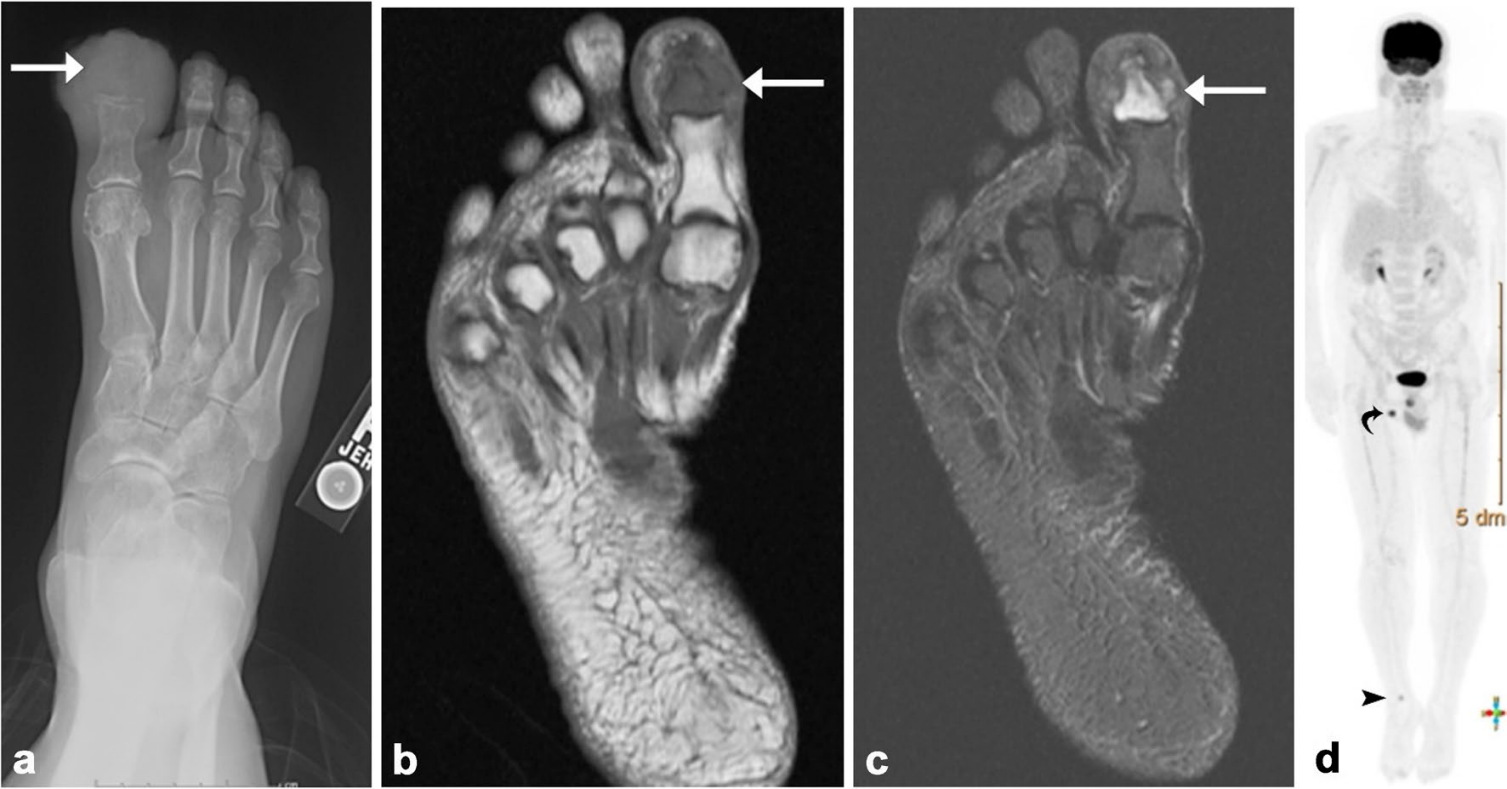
Osteosarcoma mimicking osteomyelitis. Fifty-eight-year-old male with right great toe pain. AP radiograph of the right foot (a) demonstrates an aggressive lytic lesion of the first distal phalanx (*arrow*). MR examination of the foot reveals a marrow-replacing lesion in the first distal phalanx (*arrows*), demonstrating T1 hypointensity (b) and T2 hyperintensity (c), raising suspicion for osteomyelitis. However, subsequent tissue sampling confirmed the lesion as osteosarcoma. Restaging whole body ^18^F-FDG PET-CT (d) after surgical resection and multiple cycles of chemotherapy shows hypermetabolic activity of the right ankle (*arrowhead*) and inguinal lymph nodes (*curved arrow*), with respective SUVmax values of 4.4 and 10.4, indicative of metastatic lymphadenopathy
